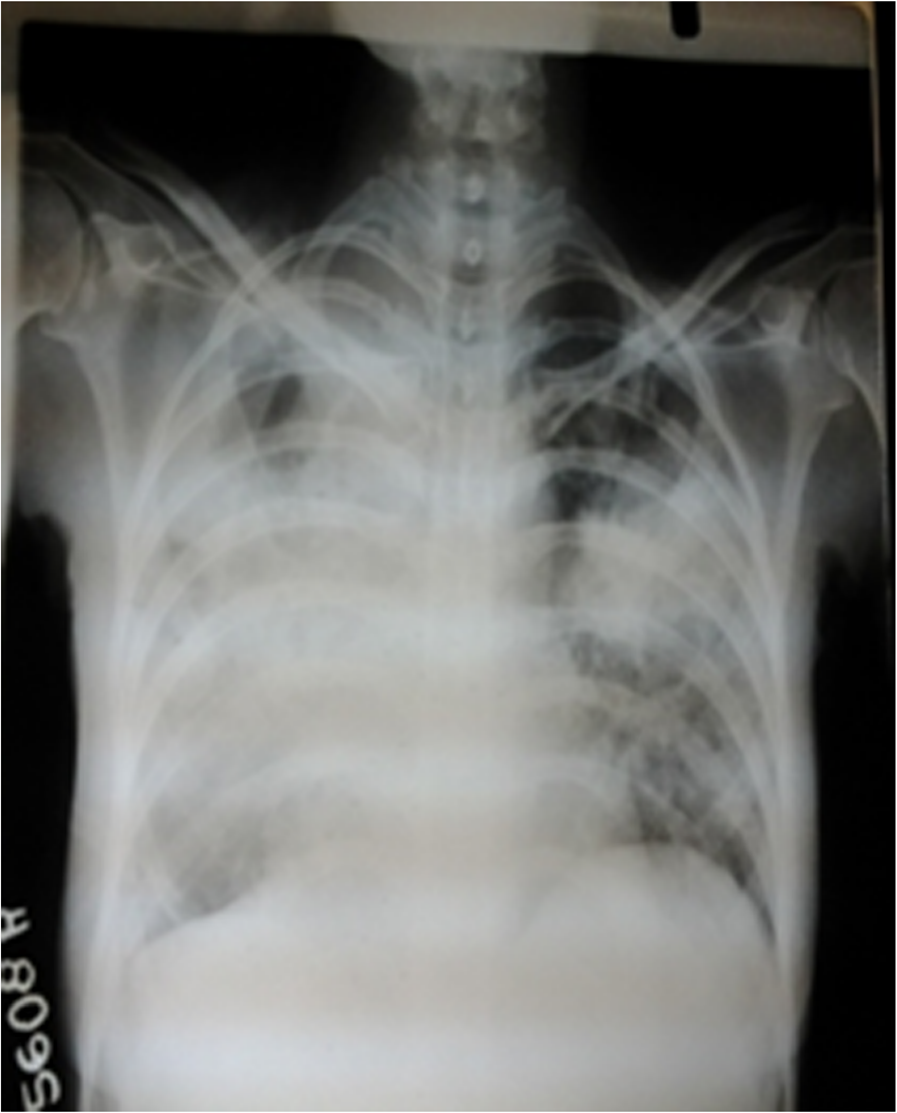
(Chest x-ray on Day 3 of admission). Extent of opacification increased with coalescing of nodules (Right > Left).
